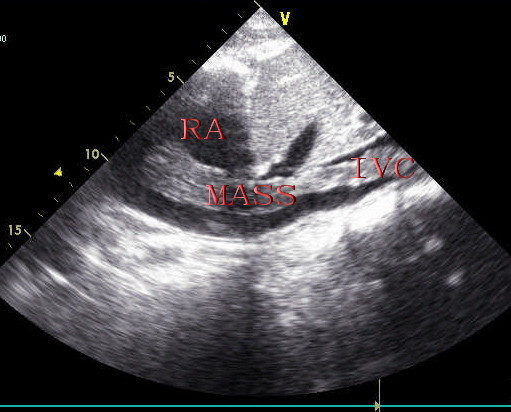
Under-ensisternum view showing mass originated from inferior vena cave, extended into right atrium and that mass of right atrium and inferior vena cave has no stalk and has well-demarcated borders with the wall of right atrium and inferior vena cave by transthoracic two-dimensional echocardiography. RA: right atrium, IVC: inferior vena cave.
